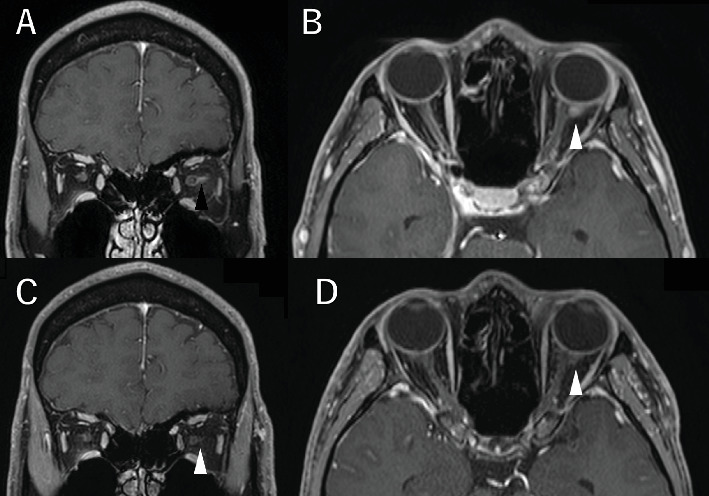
Magnetic resonance imaging (MRI) shows a mass shadow at the posterior part of the left eyeball near the macula in the orbit at the first visit: (A) T1 axial; (B) T1 coronal. On MRI 9 months later, the contrast effect of the mass shadow in the posterior part of the left eyeball has diminished. White arrowhead: orbital nodular lesion of orbital toxoplasmosis: (C) T1 axial; (D) T1 coronal with gadolinium-enhanced.
